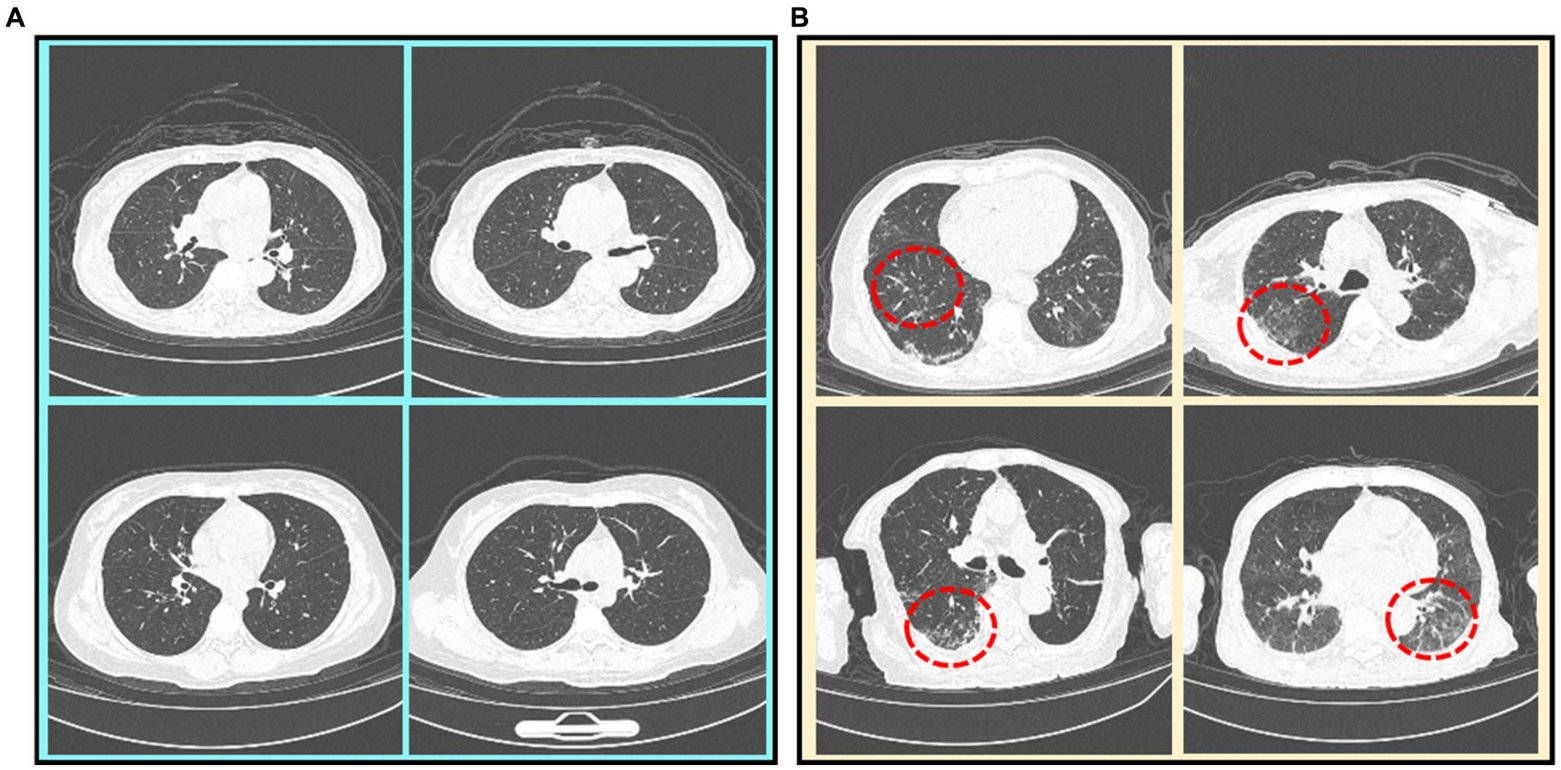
CT scan images of mild and severe Omicron pneumonia. Severe Omicron pneumonia areas are marked with red dotted circles. (A) Mild Omicron pneumonia. (B) Severe Omicron pneumonia.
